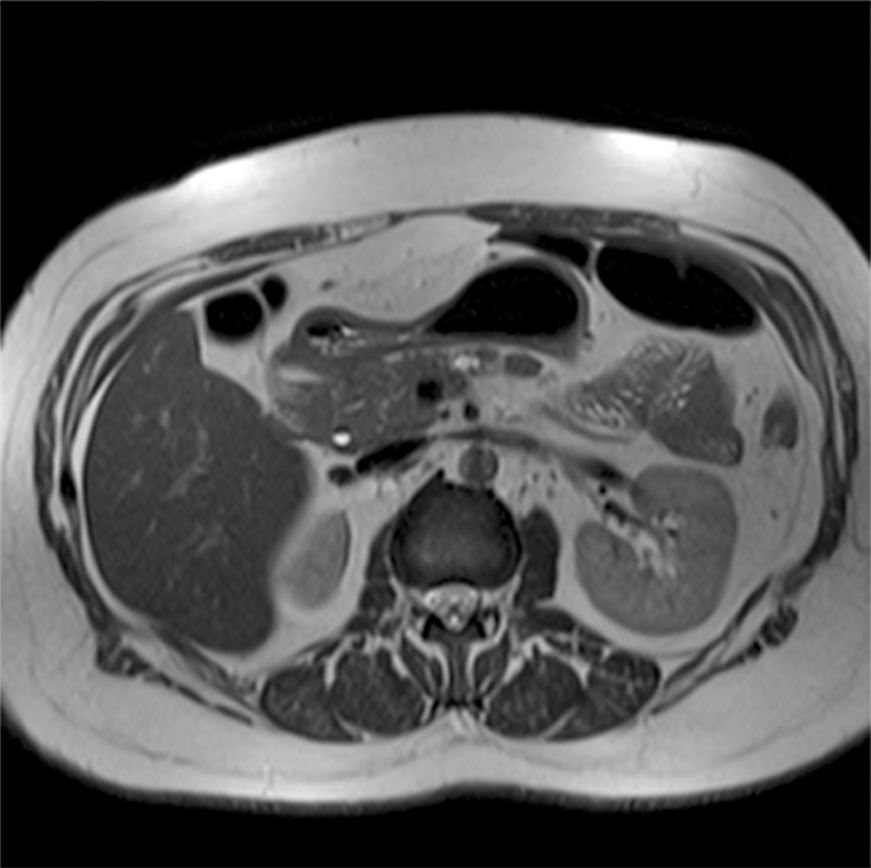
Abdominal MRI (T2-weighted sequence, axial slice), showing a lobulated cyst located in the body of the pancreas.
